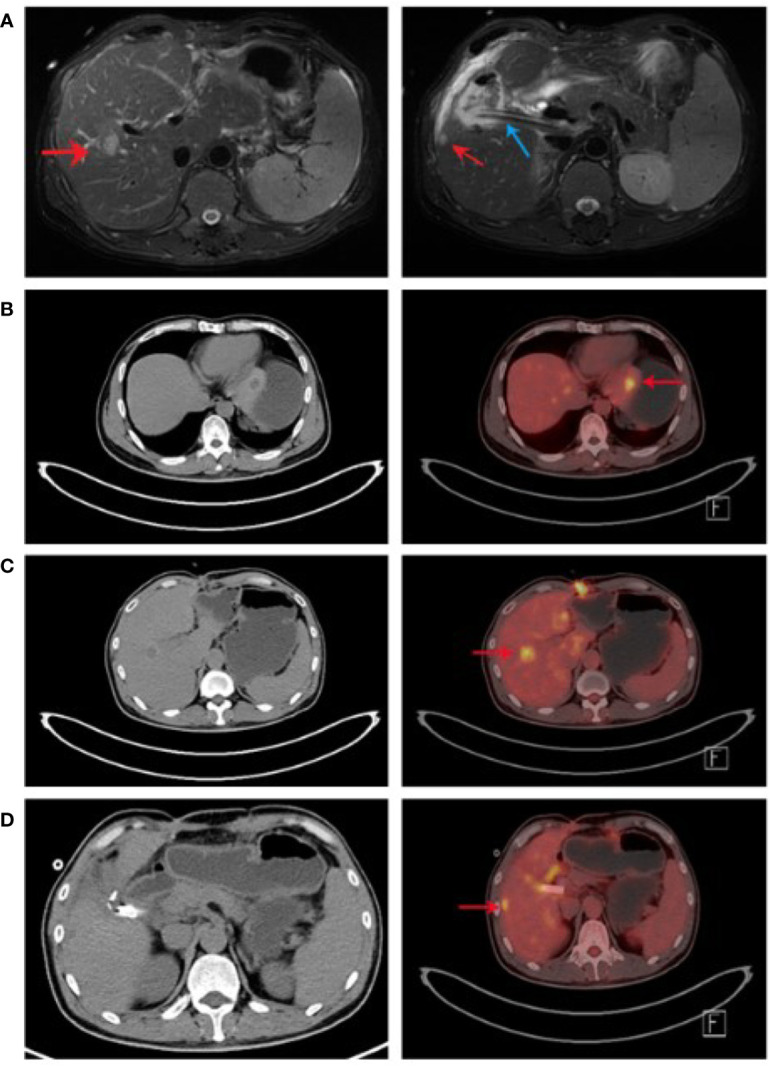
Re-examination of liver by MRI and PET-CT. (A) After the operation, liver MRI showed that the liver structure changed and metastatic nodules formed. The structure of the operation area became disordered, as shown in the shadow of the drainage tube (indicated by the blue arrow). The local signal was chaotic, and the enhancement was obvious. The remnant liverMRI of the liver had three round nodules. The larger one located in the left liver was about 1.8 cm × 1.4 cm (indicated by the red arrow in the left picture). A smaller one located in the right lobe was about 0.89 cm in diameter (indicated by the red arrow in the right picture). (B-D) PET-CT scan showed low-density nodules with sizes of 1.7 cm × 1.0 cm (B), 0.8 cm × 0.8 cm (C), and 1.5 cm × 1.4 cm (D). The nodules are indicated by red arrows.
